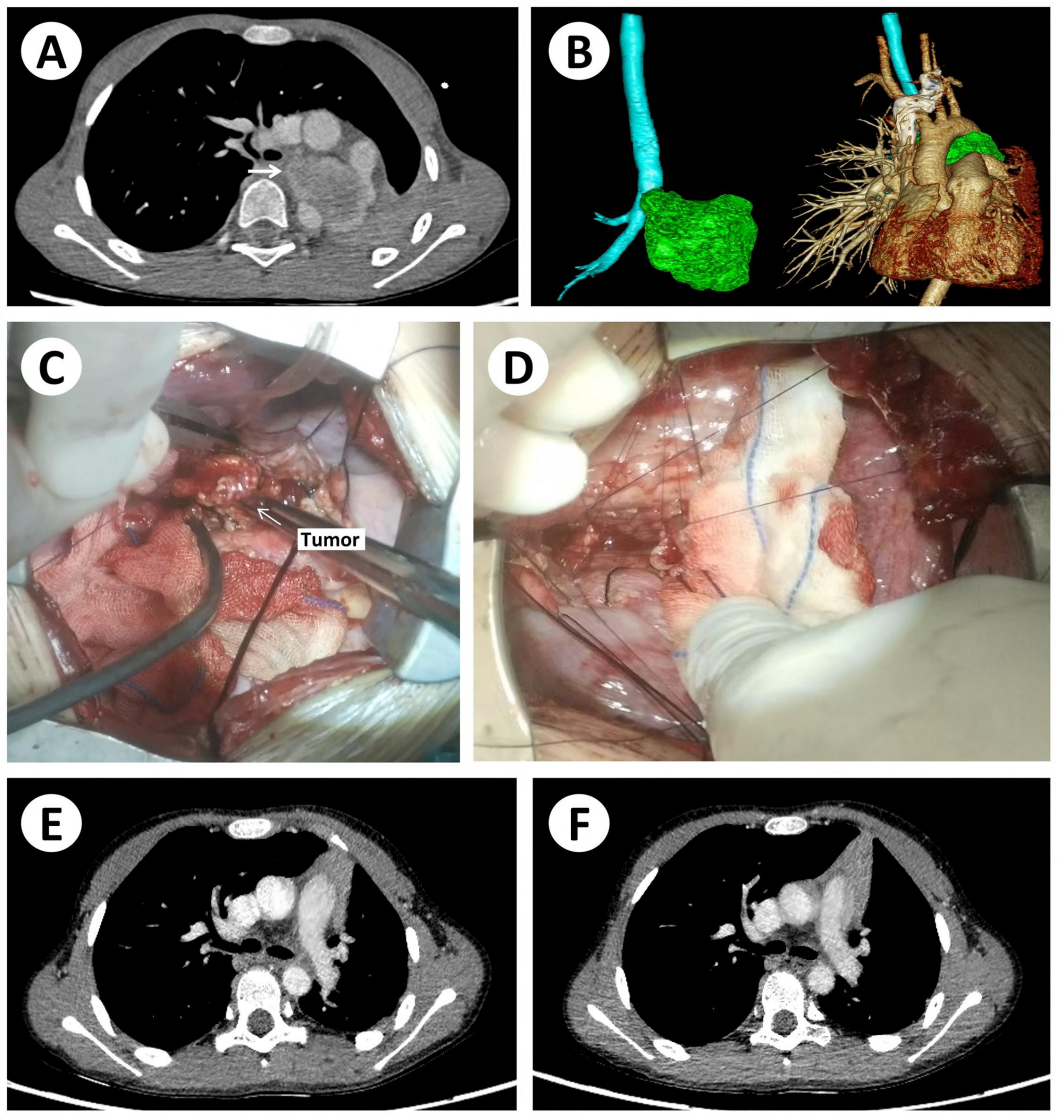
Tumor case 2, an 8-year-old boy presenting with cough. CT suggested a suspected tumor. Bronchoscopic biopsy confirmed the diagnosis of inflammatory myofibroblastic tumor, and FISH revealed ALK rearrangement. (A,B) Preoperative CT and three-dimensional reconstruction. (C) Intraoperative view showing tumor dissection and exposure. (D) Bronchial anastomosis following tumor resection. (E,F) Postoperative CT scans at 1 month and 4 months, respectively. After one month of crizotinib targeted therapy, CT showed tumor reduction, but the child developed significant dyspnea. Multidisciplinary team evaluation indicated a high likelihood of achieving surgical T0 resection. The patient subsequently underwent complete tumor resection with sleeve anastomosis of the left main bronchus, accompanied by mediastinal and hilar lymph node sampling. Continued postoperative targeted therapy was administered, and follow-up showed no recurrence.
